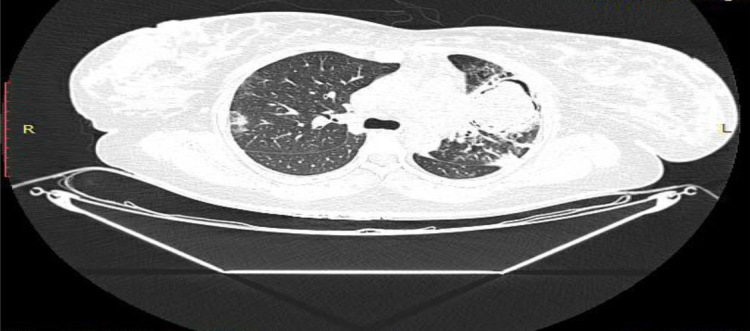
HRCT thorax suggestive of consolidation with air bronchogram and a cavity showing air crescent sign in the left upper lobe. Multiple areas of ground glass opacities were present in bilateral lung fields with a CT-severity score of 10/25 and CORADS 6.
